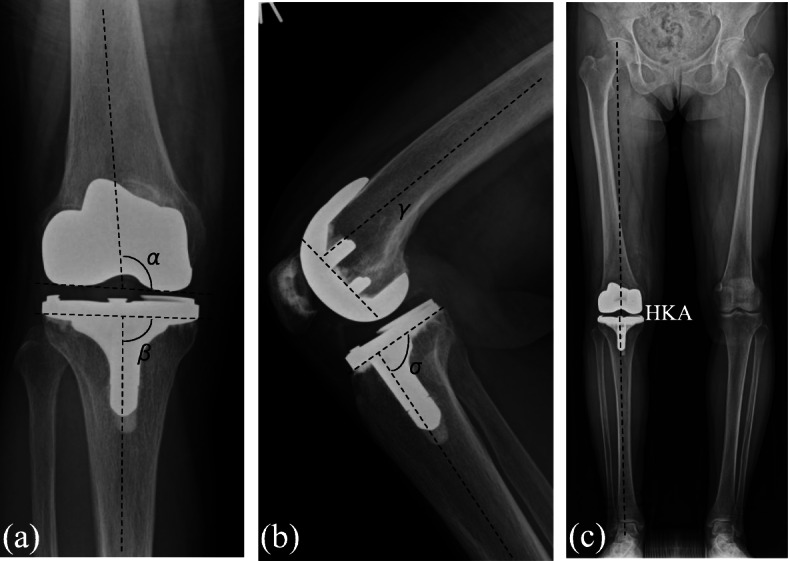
Representative (a) anteroposterior radiograph of a CR-FINE TKA showing measurement of the distal femoral valgus angle (DFVA; α) and proximal tibia varus angle (PTVA, β). b Lateral radiograph showing measurement of the femoral flexion angle (FFA; γ) and tibial slope (TS; σ). c Full-length standing radiograph showing measurement of the hip-knee-ankle (HKA) angle. Varus mechanical alignment is designated as positive for HKA angle
